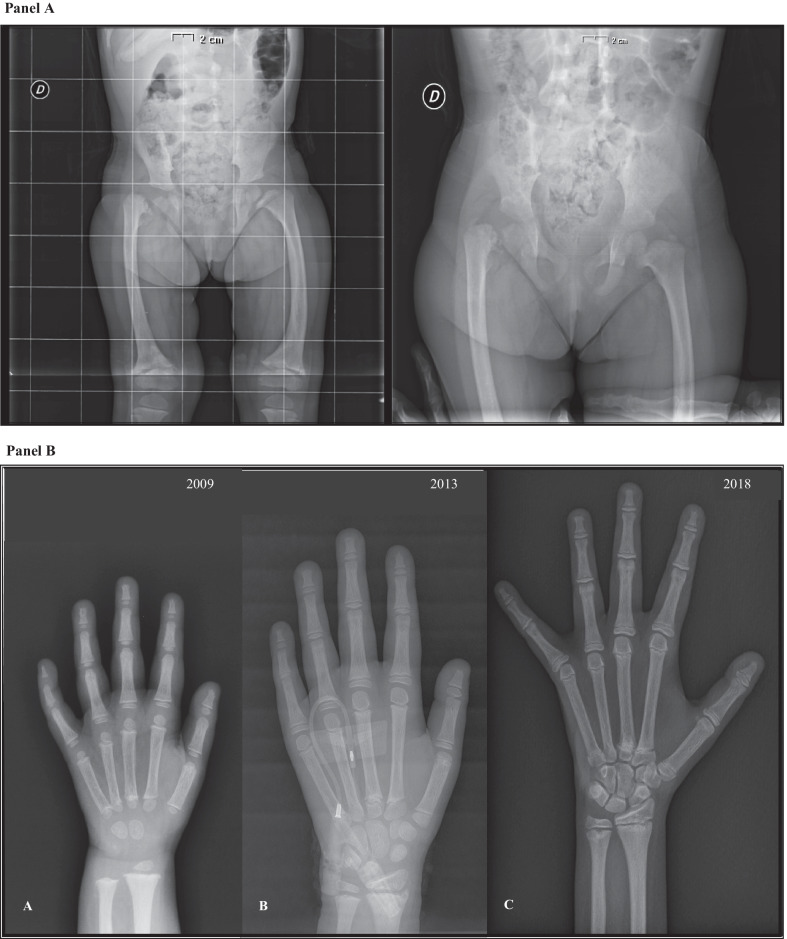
Bone abnormalities. A Pelvic X-ray of our patient evidenced right coxa vara. B Evolution of bone age through years. The progressive shape change of metacarpals that took a tubular form appears evident
